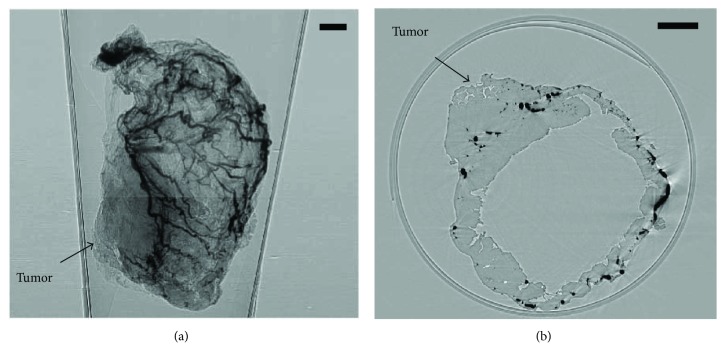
The projection and CT reconstruction images of PC-CT. (a) One projection image; (b) one CT slice (bar: 1 mm).
